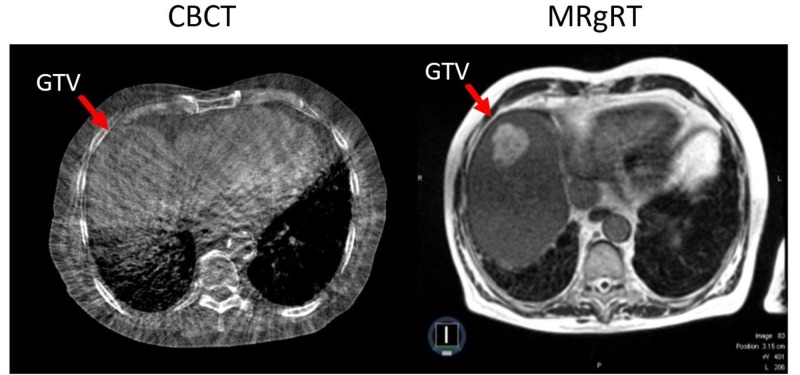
GTV localized with CBCT (left) and MRIdian MRgRT setup scan (right) for fraction 2 and fraction 1, respectively, for patients undergoing liver stereotactic body radiation therapy for hepatocellular carcinoma. GTV: gross target volume; CBCT: cone-beam CT; MRgRT: magnetic resonance-guided radiation therapy
